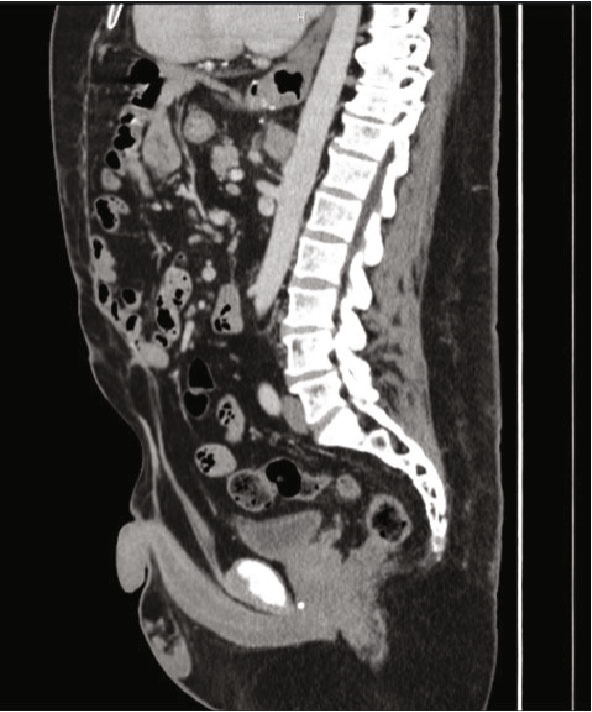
Sagittal CT demonstrating high bladder neck and abnormal penoscrotal anatomy.
